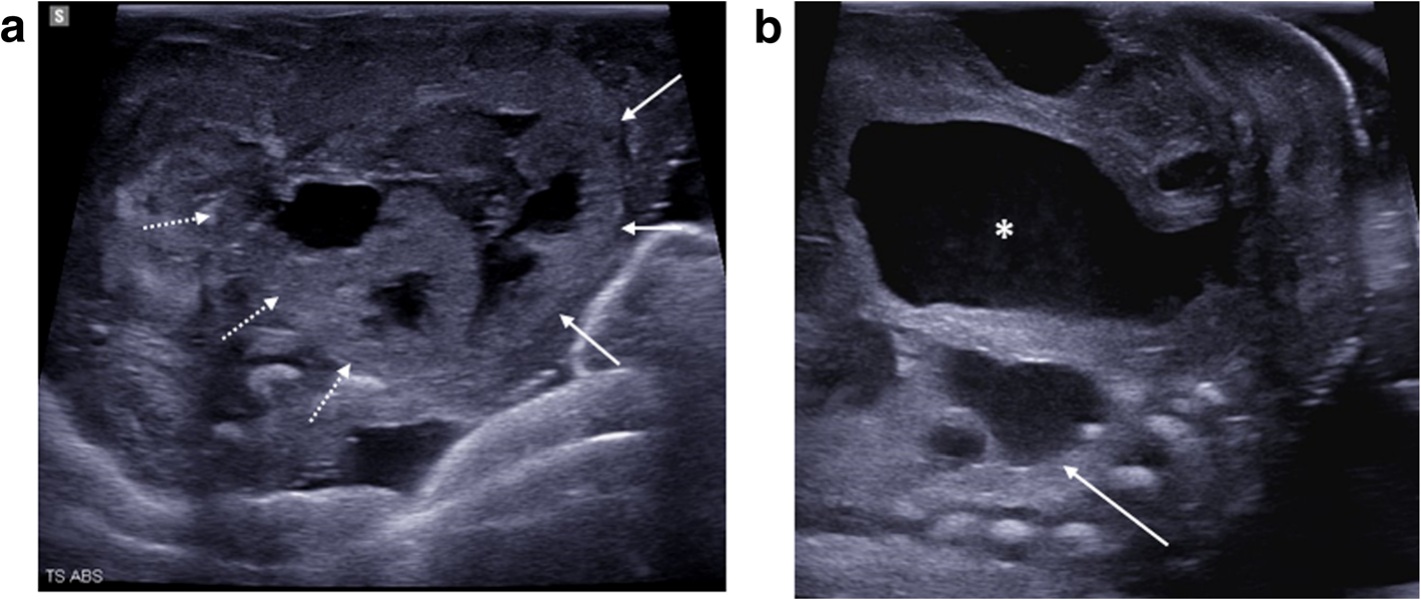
Cross-fused renal ectopia (‘pancake kidney’) in a foetus at 22 weeks gestation, following termination of pregnancy for multiple congenital anomalies. The post-mortem ultrasound images were acquired 10 days after delivery. Transverse ultrasound images through the lower abdomen (a) demonstrate cross-fused kidneys. The right moiety (dotted arrows) and left moiety (solid arrows) are demonstrated and show moderate calyceal dilation. On the sagittal ultrasound imaging of the distended urinary bladder (asterisk) (b), one can partially visualise the kidneys, demonstrating their pelvic location in the abdomen (solid arrow).
